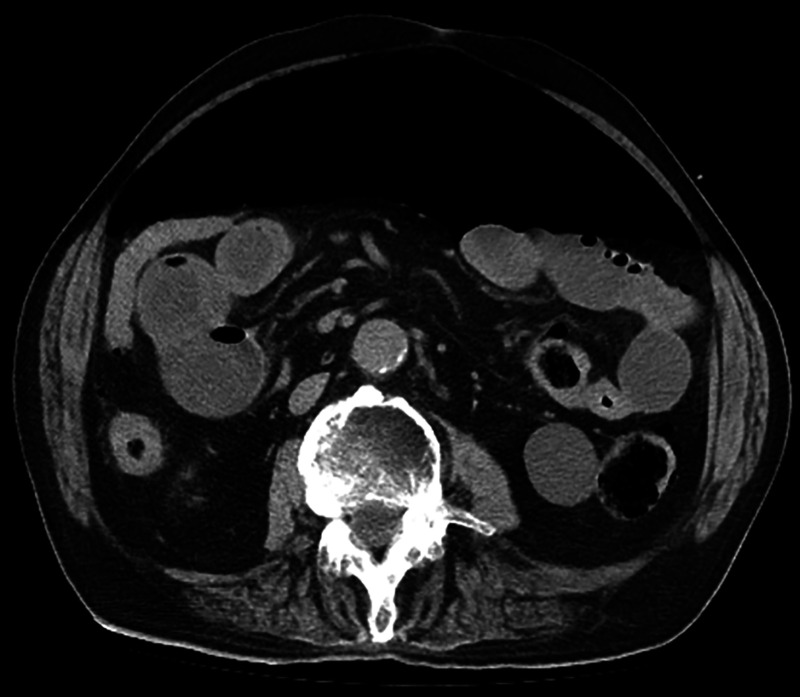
Enhanced computed tomography (CT) reveals a large amount of free air in the abdomen without any signs of gastrointestinal perforation.
